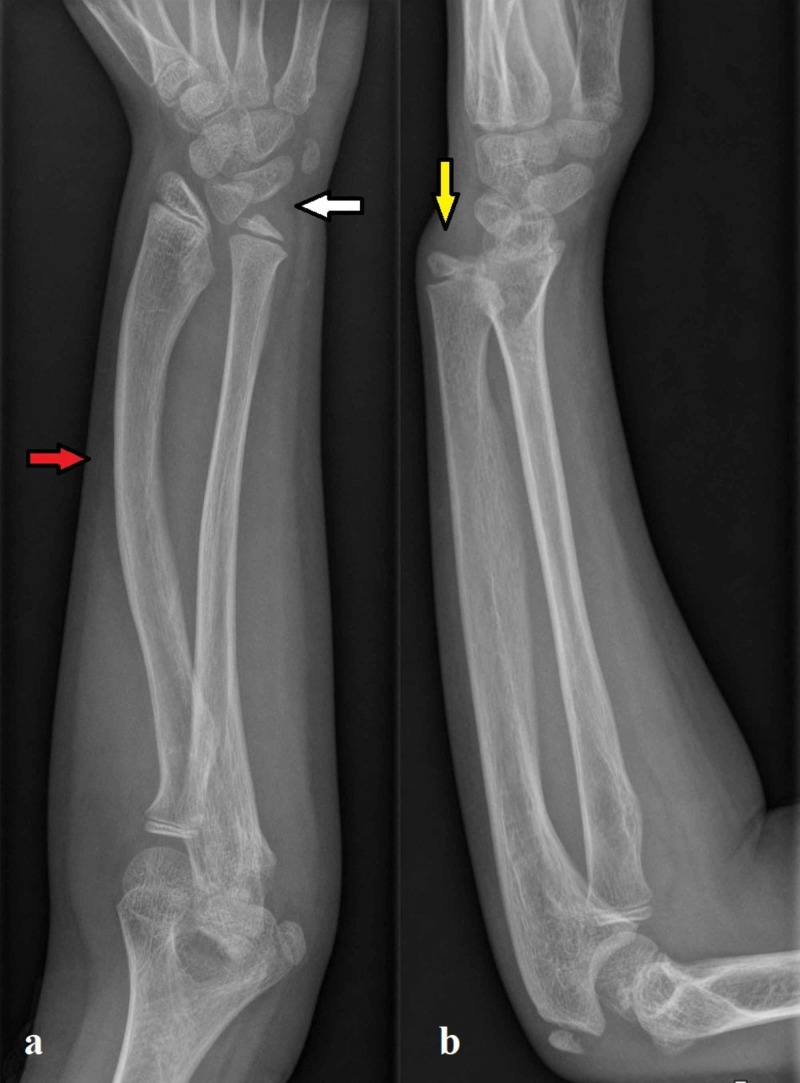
Radiography. The anteroposterior radiograph (a) of the left wrist shows an increased radial inclination (~ 32°) with the deformation of the carpus that acquired a triangular appearance (white arrow). Shortening and bowing of the radial diaphysis (red arrow) are also evident. The lateral radiograph (b) shows the volar tilt of the distal radius with an angular measure at the upper limit of normality (~ 21°). Dorsal subluxation of the ulnar head (yellow arrow) is also present.
